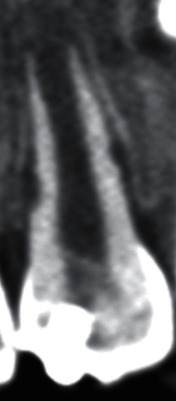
Coronally sectioned CBCT image indicating evidence for external root resorption.
